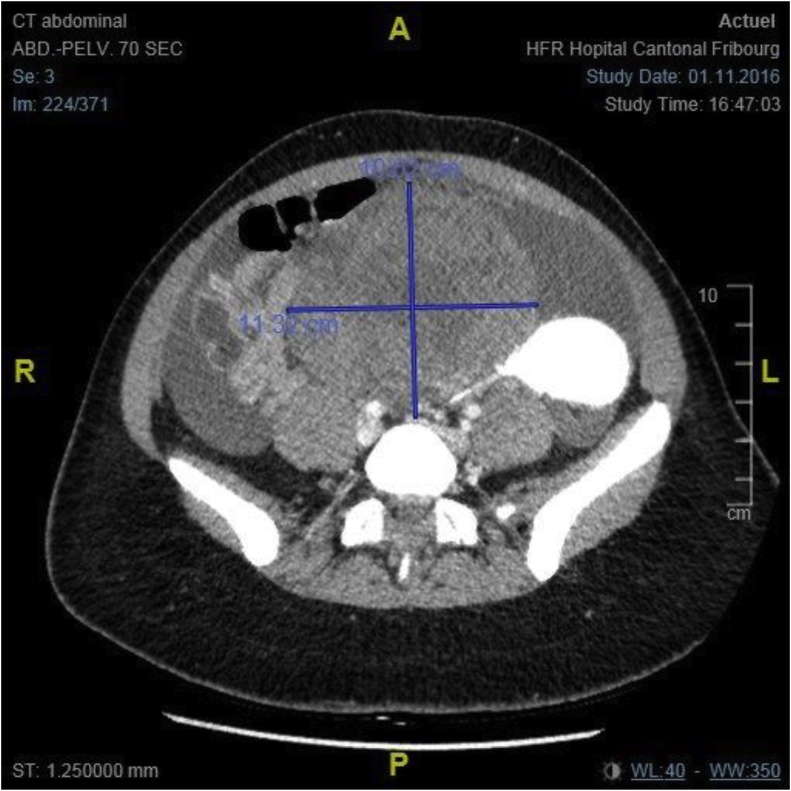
The CT Scan shows a voluminous pelvic mass of 98 × 112 × 125 mm attached to the uterus, with heterogenous enhancement and probably growing from the left ovary.
